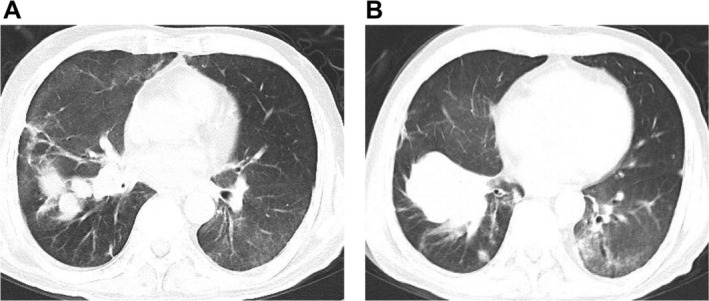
Chest CT on 20 September 2020. The soft tissue mass shadow within the bronchus of the right lower lobe and the shadow of solid changes in the distal lung tissue have both decreased in size compared with previous assessments.
